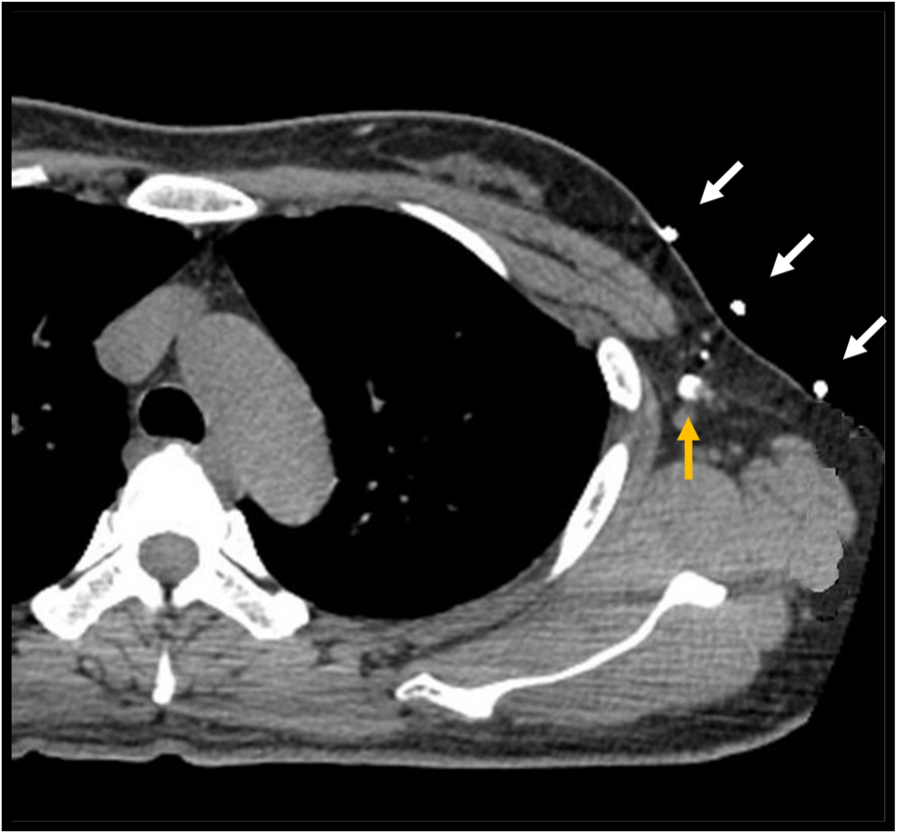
Sentinel node (yellow arrow) identified by axial computed tomography with localizing marker (white arrows).
